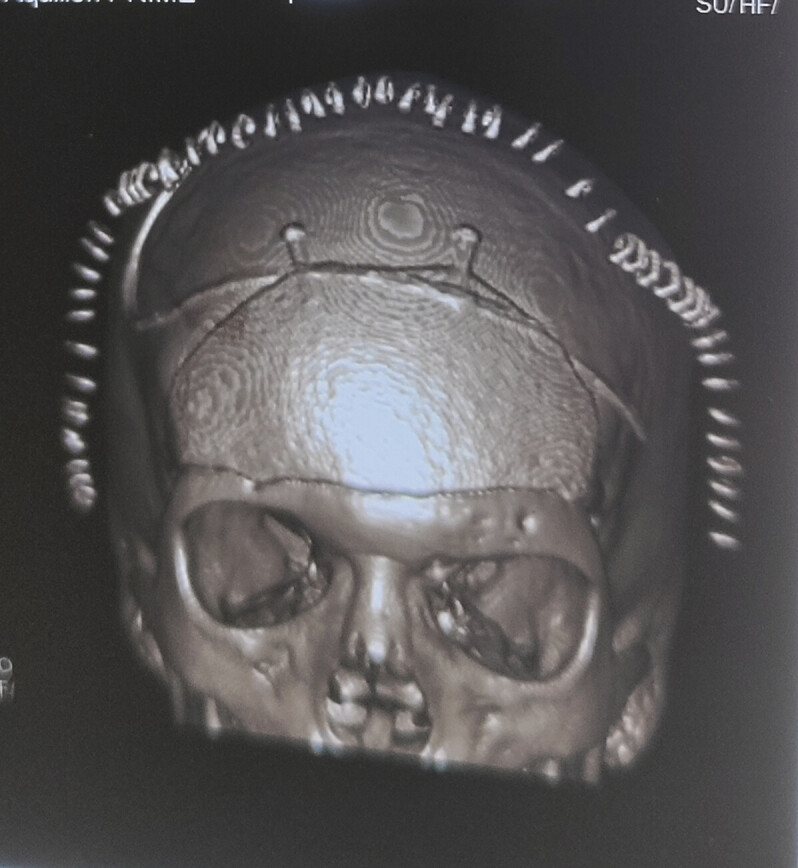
Postoperative computed tomography (CT) of brain three-dimensional reconstruction image after titanium precontoured implant cranioplasty, showing complete closure of the previous craniectomy defect.
